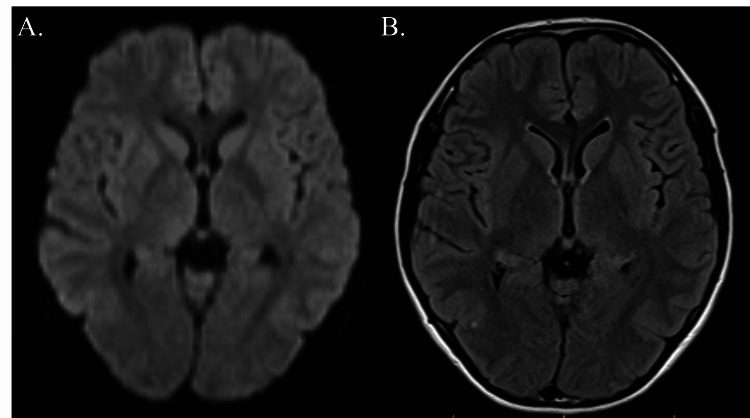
Brain magnetic resonance imaging (MRI) performed on admission (A) Diffusion-weighted MRI and (B) fluid-attenuated inversion recovery (FLAIR) MRI showing no remarkable findings
